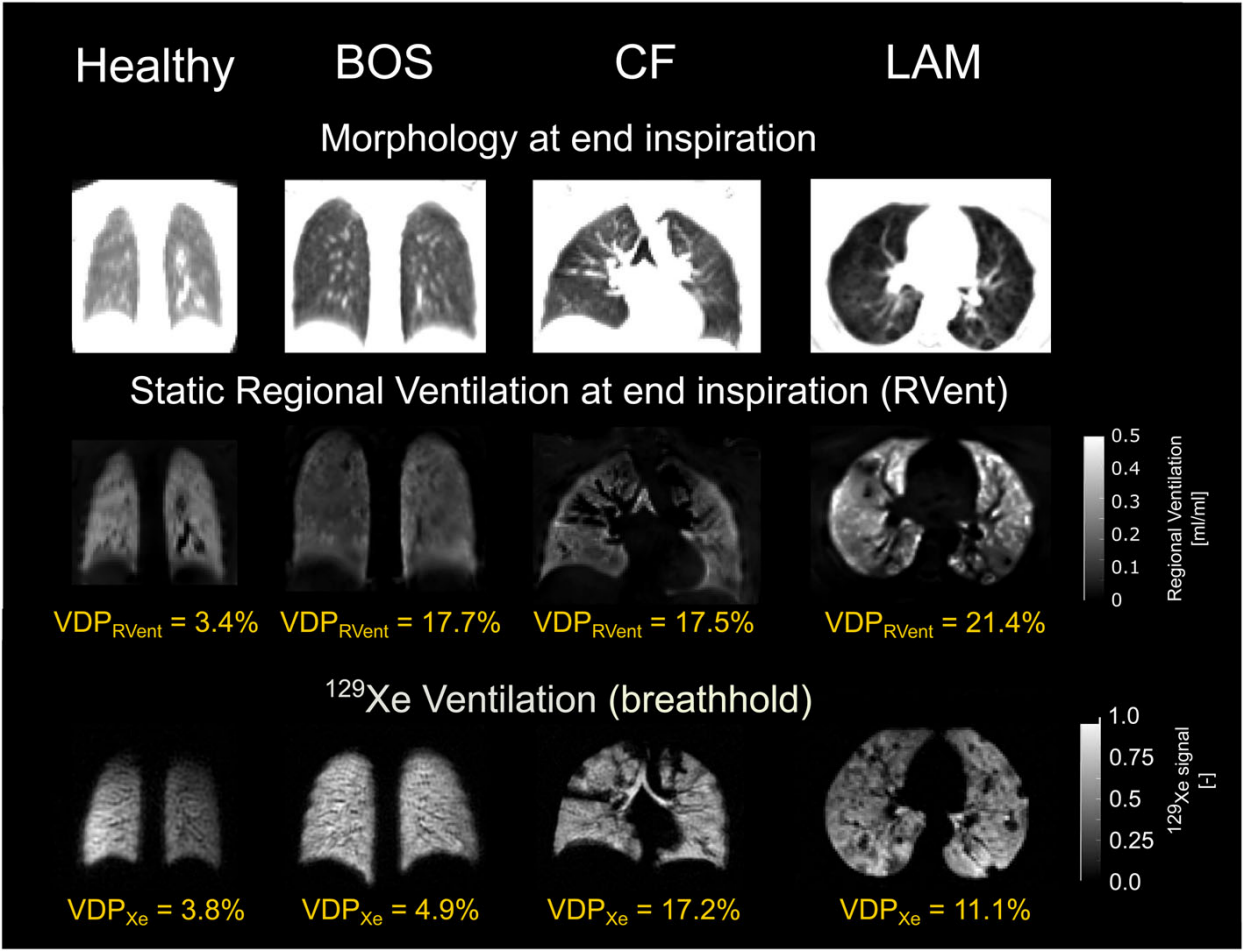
Comparison of static three‐dimensional PREFUL (phase‐resolved functional lung) FLORET (fermat‐looped orthogonally encoded trajectories) ultrashort echo time MRI (*first–second row*) and breath‐hold ^129^Xe ventilation MRI (*third row*). Four study participants are depicted: healthy volunteer (*first column*, female, 13 years old), subject with bronchiolitis obliterans syndrome (BOS; *second column*, male, 24 years old), subject with cystic fibrosis (CF; *third column*, female, 25 years old), and subject with lymphangioleiomyomatosis disease (LAM; *fourth column*, female, 39 years old). For both measurements, the whole‐lung ventilation defect percentage (VDP) values are listed below the respective ventilation map.
